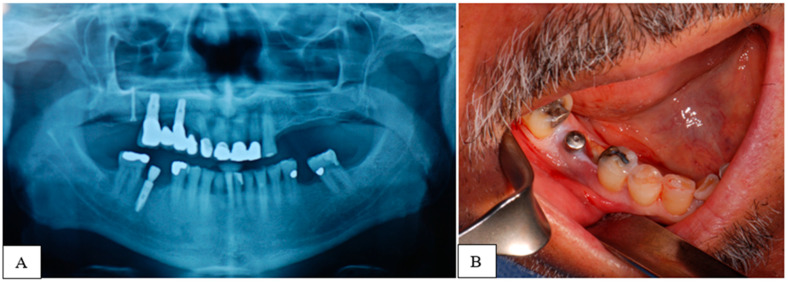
(A) Clinical case BRONJ stage 1b with osteonecrosis in the right mandible. Preoperative radiograph (T0). (B) Intra-oral clinical picture (T0) with distal bone exposure at second lower right premolar.
